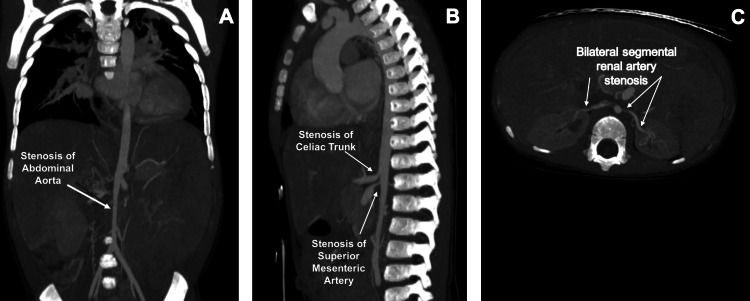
CT angiography of the chest, abdomen, and pelvis. The images show (A) coronal view of stenosis of the abdominal aorta, (B) sagittal view of stenosis at the origin of the celiac trunk and superior mesenteric artery with marked post-stenotic dilatation, and (C) axial view of bilateral segmental renal artery stenosis.
